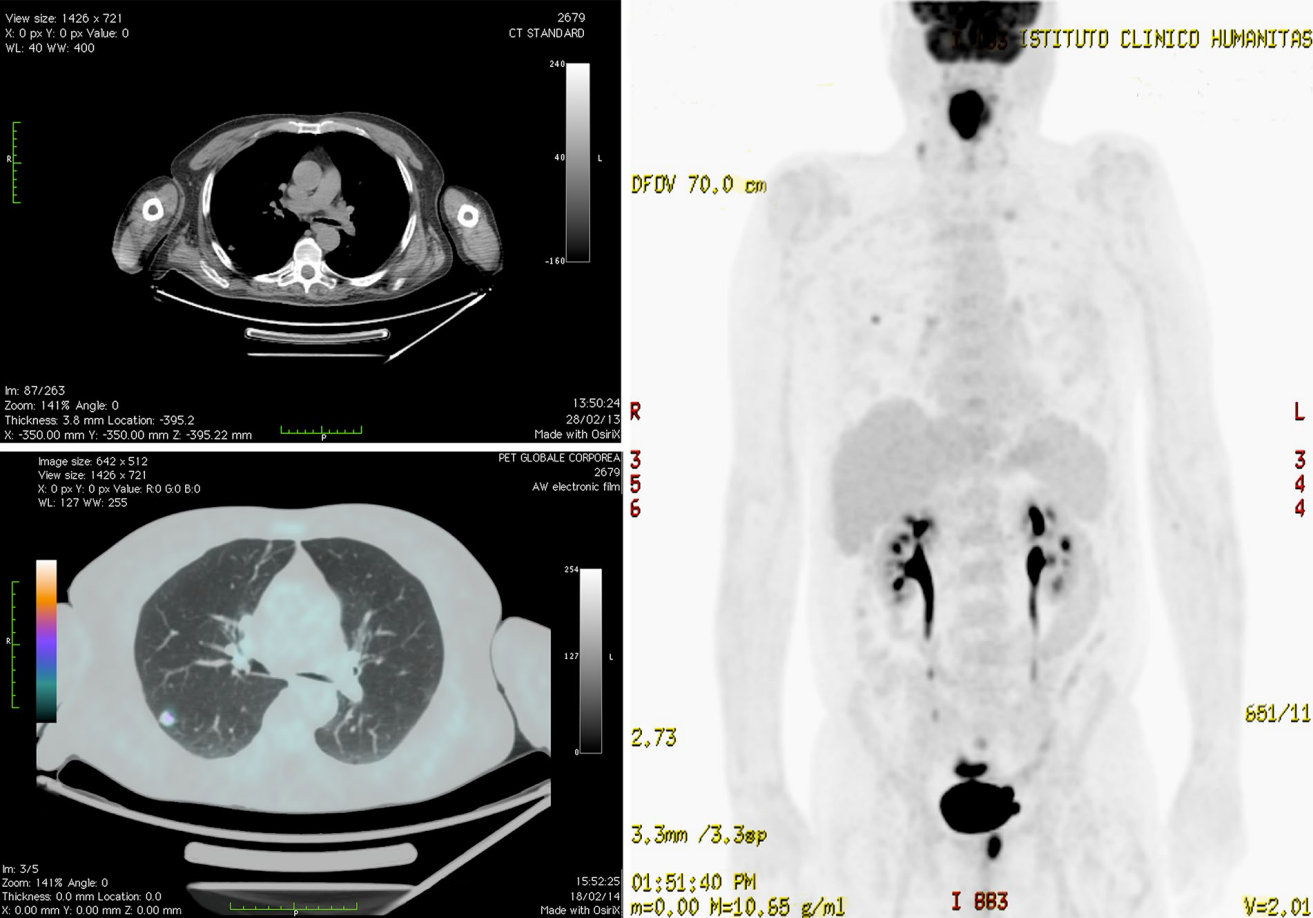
Top left panel* Axial non-contrast CT scan shows no significant mediastinal, hilar and axillary lymphadenopathy. *Bottom left panel* Axial non-contrast lung window setting CT shows a solid nodule at the upper segment of right lower lobe. *Right panel* Coronal view whole-body PET scan shows focal uptake of at the upper segment of right lower lobe, sigmoid colon and prostate gland
